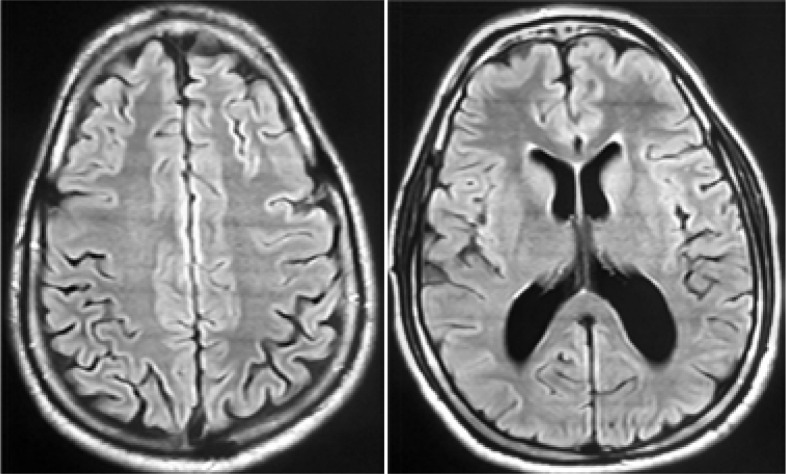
End-of-therapy MRI, without abnormalities
